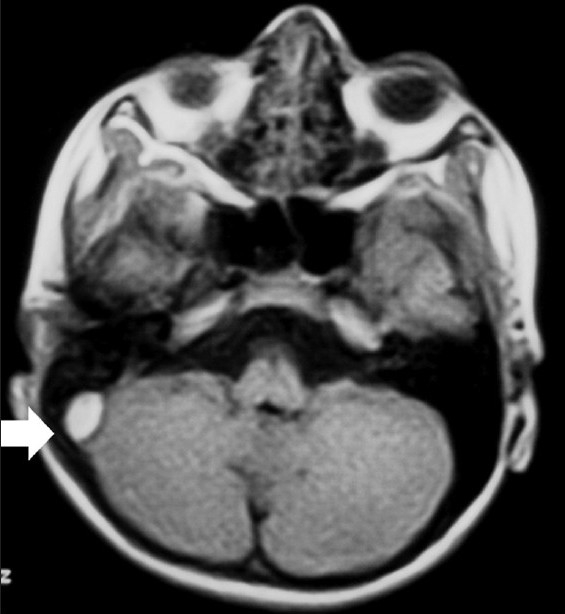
MRI T1W image showing iso- to hyperintense area in the region of right sigmoid sinus (arrow)
